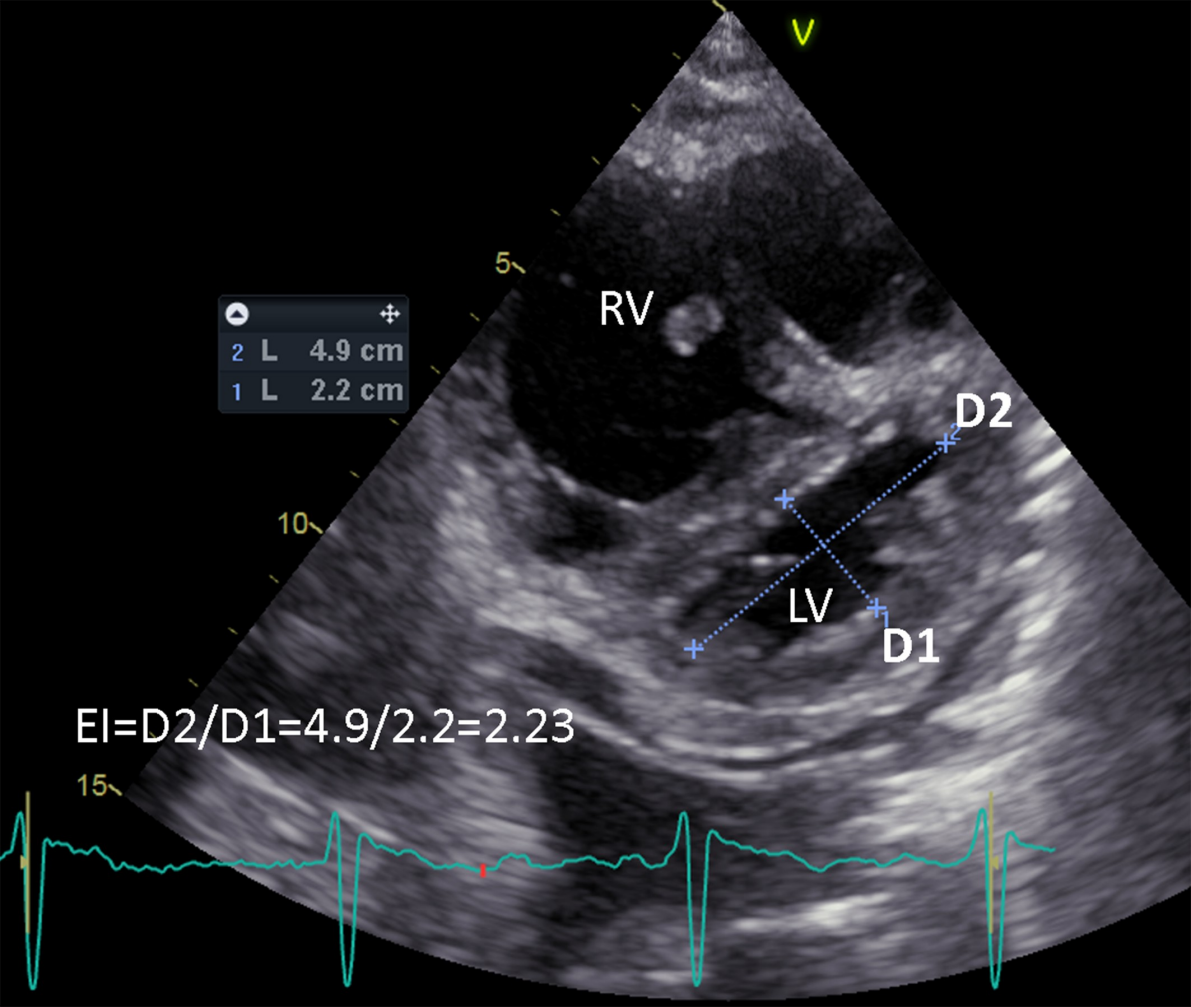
Measurement of echocardiography-derived Eccentricity Index (EI) at end-systole. EI is defined as the ratio of D2/D1, where D1 is the left ventricle short-axis diameter perpendicular to the septum and D2 is the left ventricle short-axis diameter parallel to the septum. LV, left ventricle; RV, right ventricle.
